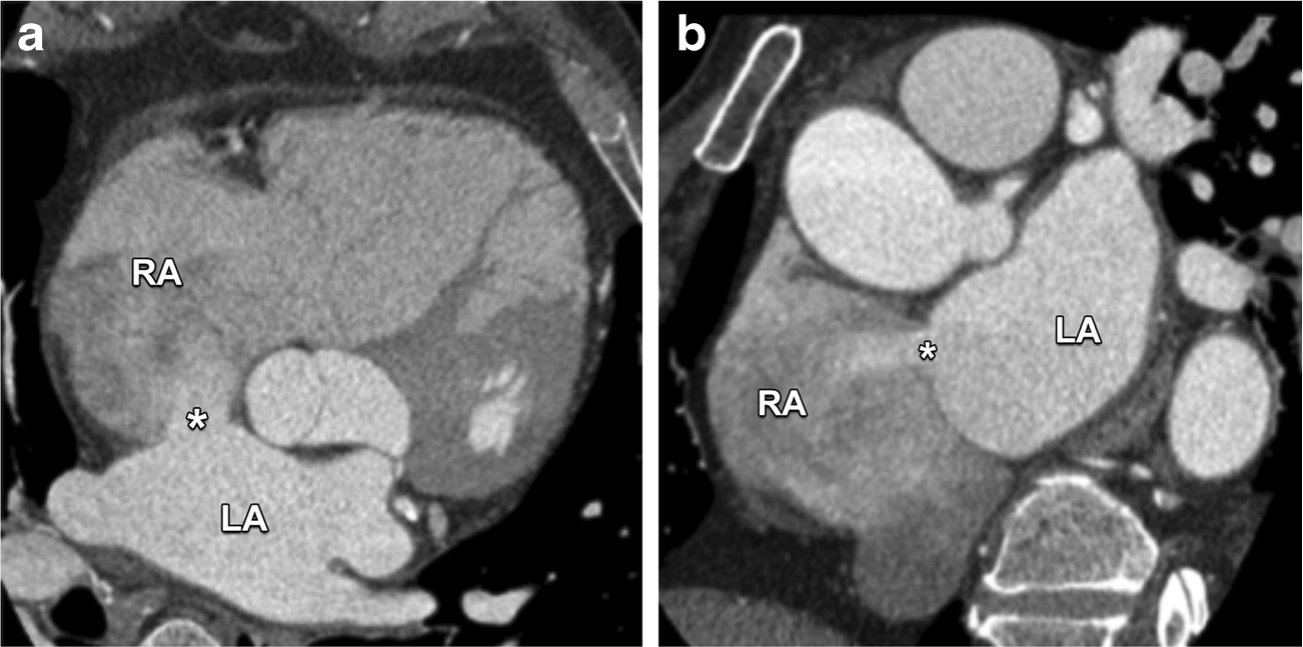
Axial (a) and coronal (b) contrast-enhanced CT images clearly depict an ostium secundum defect (*asterisk*) at the fossa ovalis with shunting of contrast-enhanced blood from the left atrium (LA) to the right atrium (RA). Contrary to a patent foramen ovale, the flow is perpendicular to the axis of the interatrial septum because of the absence of an overlapping septal flap. In large defects like in this case, this can lead to volume overload and dilatation of the right atrium and ventricle
